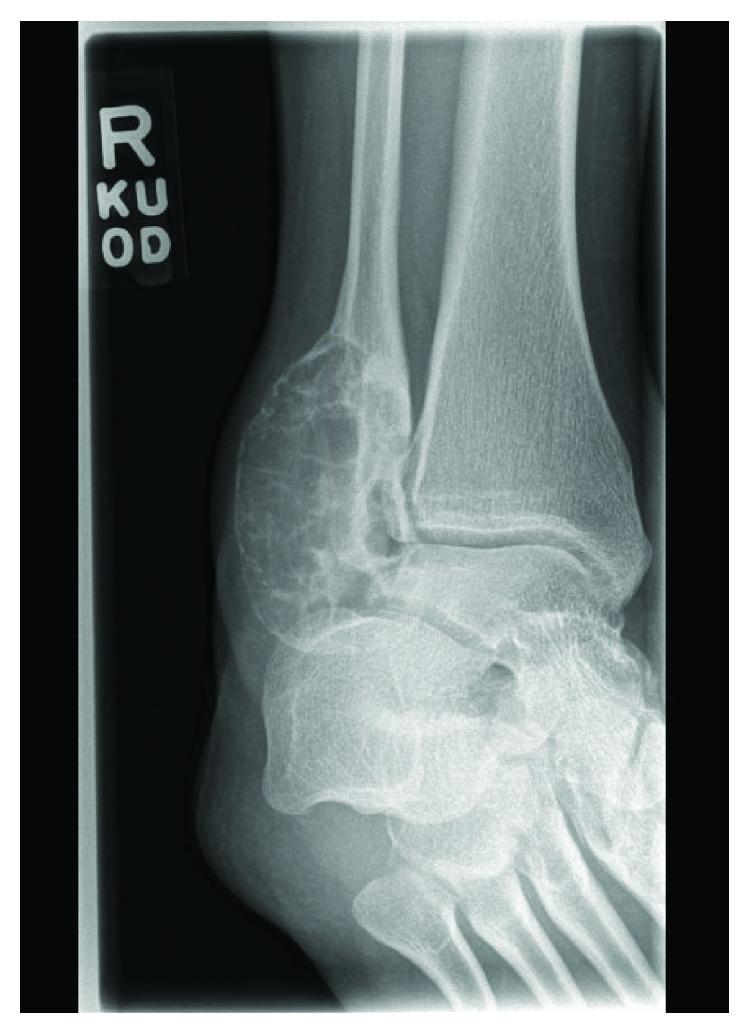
Pretreatment with denosumab: mortise radiograph showing a large lytic, expansile bone lesion with cortical disruption.
